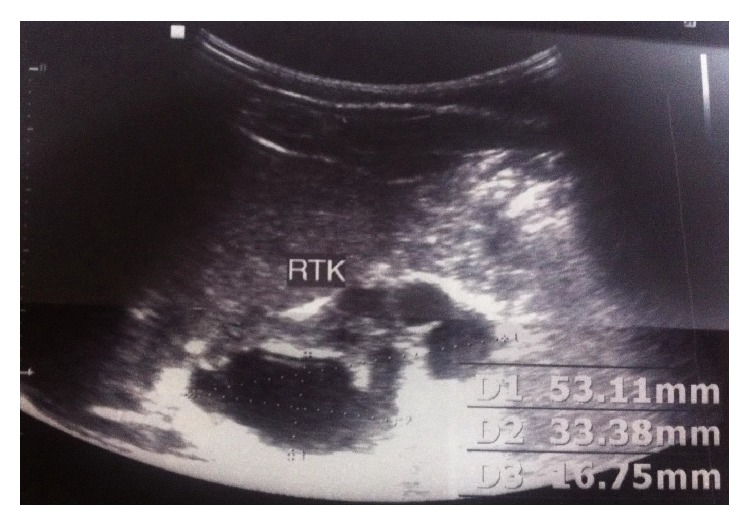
Sonography was done in 6th day which shows a right dysplastic kidney with the largest cyst size measuring 33∗16 mm after cyst aspiration.
